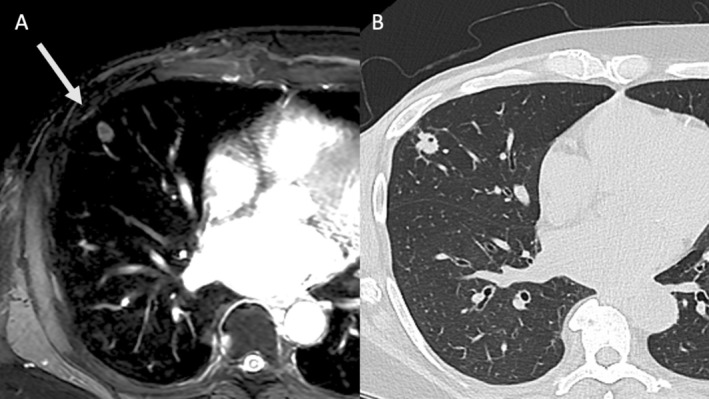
Lung metastasis from a moderately differentiated ductal adenocarcinoma of the pancreas with mucinous differentiation (arrow). (A) The T1/T2‐weighted balanced steady‐state free precession (bSSFP) acquisition (TR 3.61, TE 1.5, TI 200, BW 434.02, 1.5 T field) shows a well‐defined lesion in the lateral segment of the middle lobe. (B) CT image of the same lesion.
